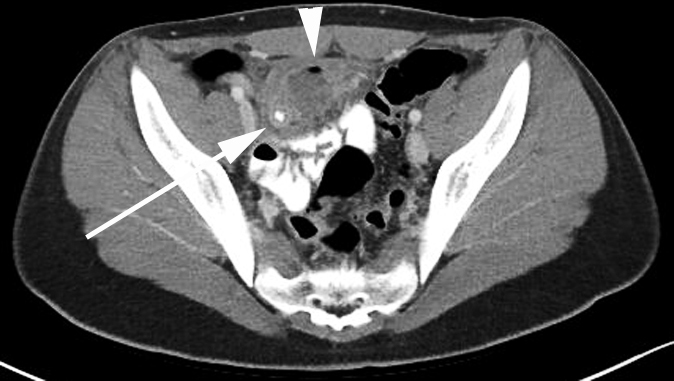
A 14-year-old male with abdominal pain who was diagnosed with complicated appendicitis (same patient as Figure 3). Axial CT image demonstrates an appendicolith within the lumen of the dilated appendix. There is a surrounding air and fluid containing collection consistent with an abscess.
